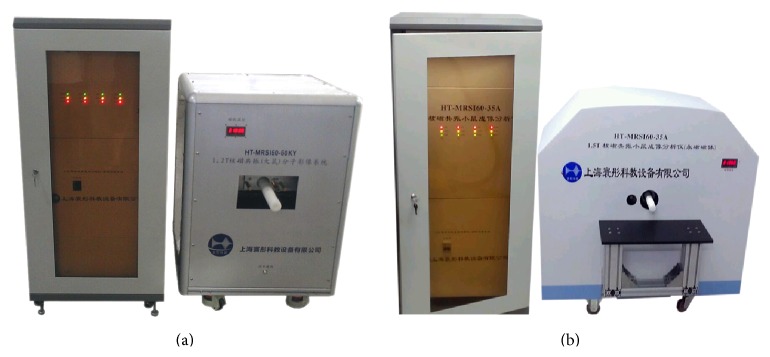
MRI systems with 1.2 T (a) and 1.5 T (b) permanent magnetic circuits.
